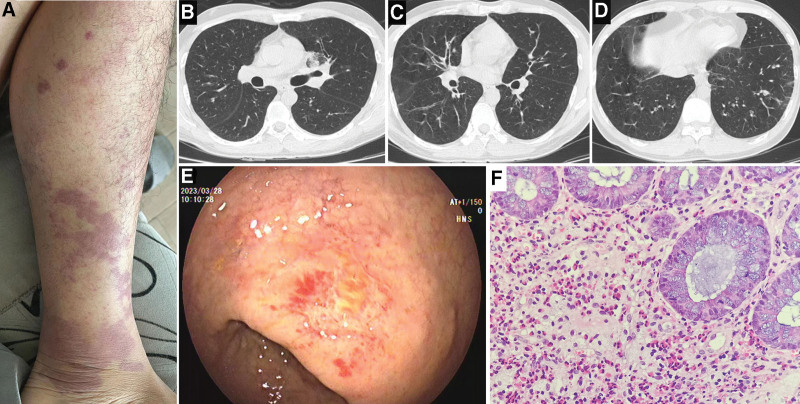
(A) The patient presented multiple patchy rashes on both lower limbs; the rashes were dark red in color, with no tenderness, no itching, no nodules, and no blisters. (B–D) Chest CT showed bilateral ground-glass opacities, small nodules, and bronchiectasis. (E) The gastrointestinal endoscopy diagnosis was chronic superficial gastritis and multiple rectal ulcers. (F) The pathological diagnosis was focal (rectal) infiltration of numerous eosinophils (HE, ×400). CT = computed tomography.
